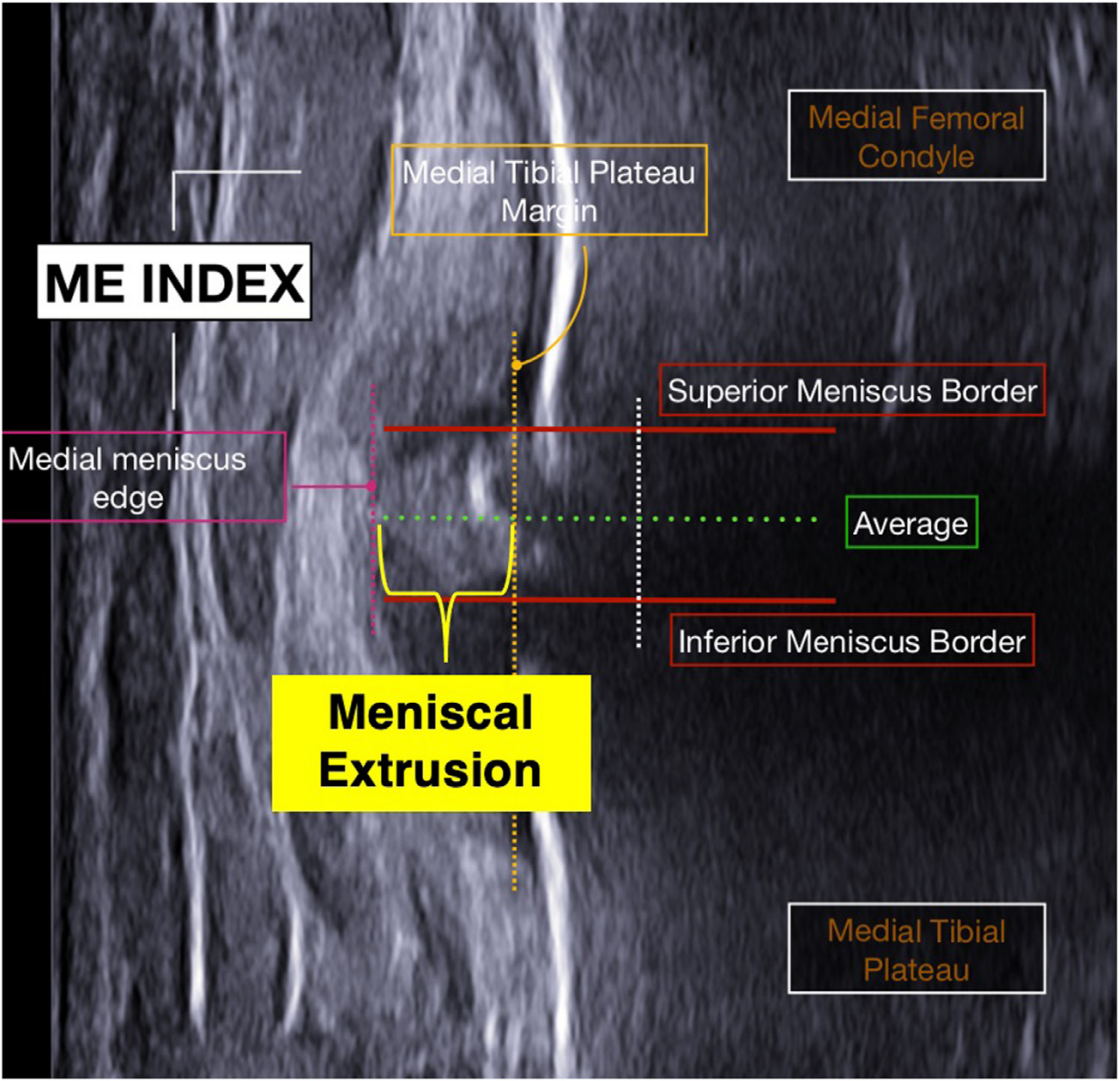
Once the probe is positioned over the posterior border of the MCL in the long-axis plane on the ultrasound image, we can begin making ME measurements. Draw a line parallel with the cortex of the proximal tibia at the articular margin. This will serve as a reference line and represent 0 millimeters of ME. Next, 2 lines at the superior and inferior meniscal borders should be drawn, with a third line going down the middle, denoted by “Average.” Lastly, a line should be drawn at the medial meniscal edge. The distance from the reference line and edge line will be the final meniscal extrusion measurement. (MCL, medial collateral ligament; ME, meniscal extrusion.)
